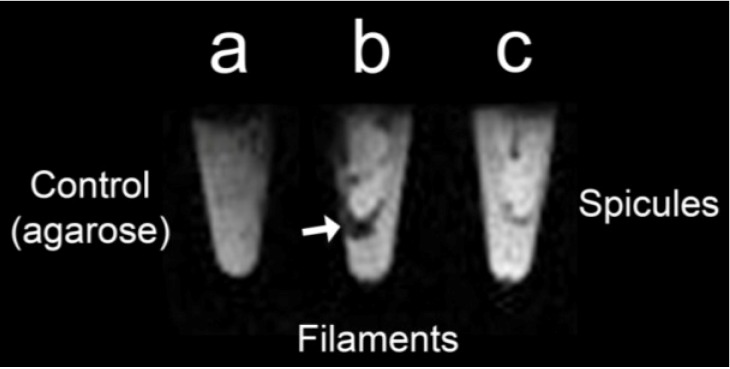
Magnetic Resonance Imaging (MRI) analysis of skeletal elements of *S. domuncula* immobilized into an agarose gel (1% w/v)*.* (a) Agarose gel (1% w/v) was used as the control, showing no signal; (b) axial filaments from *S. domuncula* show strong signals (dark spots) derived from T2*-weighted from the iron oxide agglomerates co-localized within the axial filaments (arrows); (c) Cleaned spicules do not show any signal/contrast.
